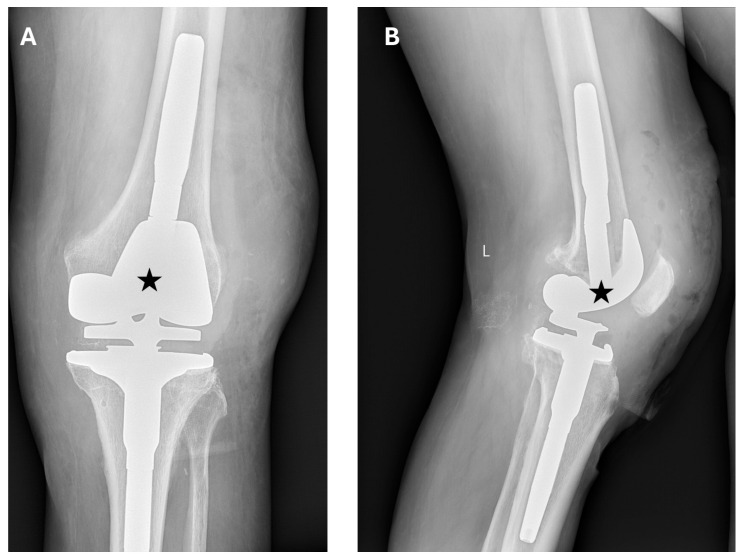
Postoperative X-ray—1 day after R-TKA. (A) Anteroposterior (AP) plane. (B) Lateral plane. The correct positioning of the prosthesis components (black asterisk) is visible.
